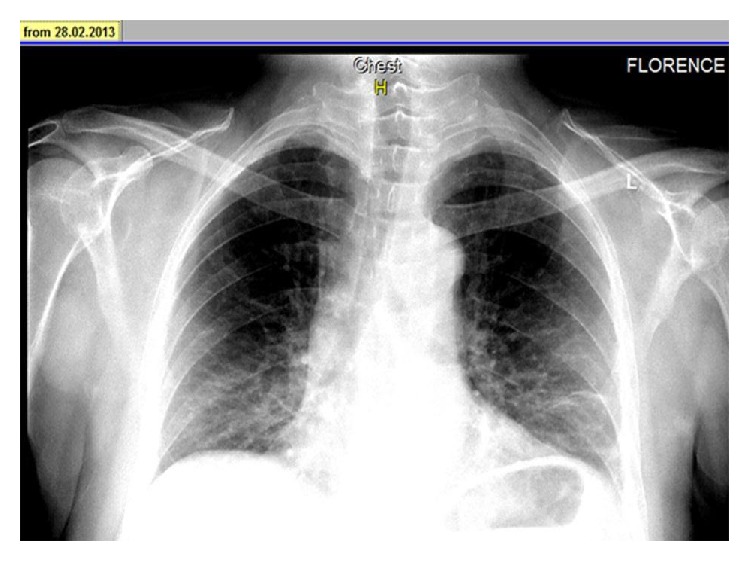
PA radiography showed linear densities in the left lower zone and elevation of the left diaphragm.
